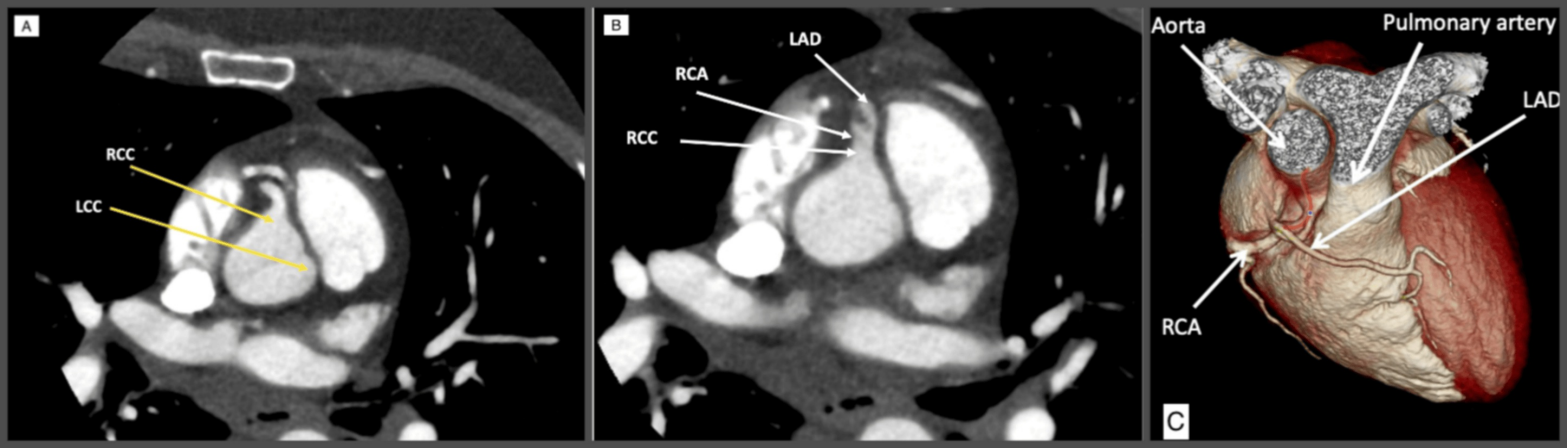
Computed tomography coronary angiography shows a single coronary artery originating from the right aortic sinus. LAD: left anterior descending artery; RCA: right coronary artery; LCC: left coronary cusp; RCC: right coronary cusp
